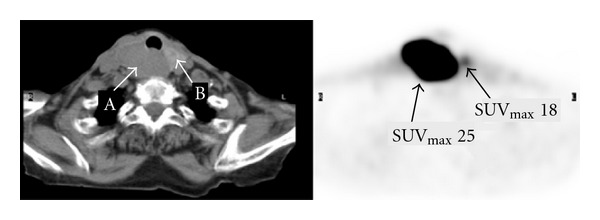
Transaxial FDG PET-CT images of the neck show a large lesion with SUV_max⁡_ 25 in the right lobe of the thyroid (arrow A). There is a 1.5 cm nodule with mild to moderate uptake in the left lobe, but unexpectedly measured SUV_max⁡_ is 18 (arrow B).
